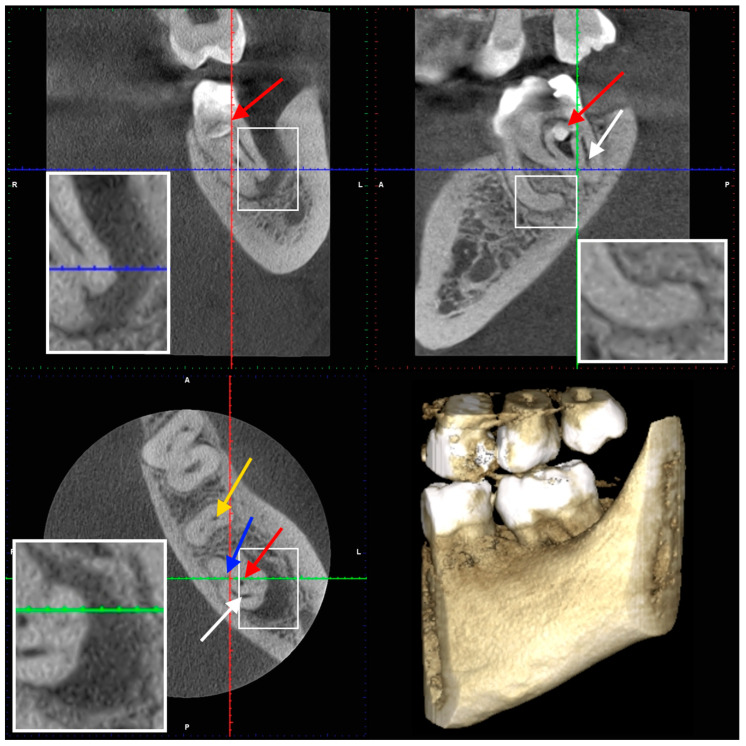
Tooth 3.7—CBCT scans (the second appointment): yellow arrow—mesial canals, blue arrow—two distal canals, white arrow—semicircular space, red arrow—the additional tooth. The magnification of apical regions are visible in the zoomed areas.
